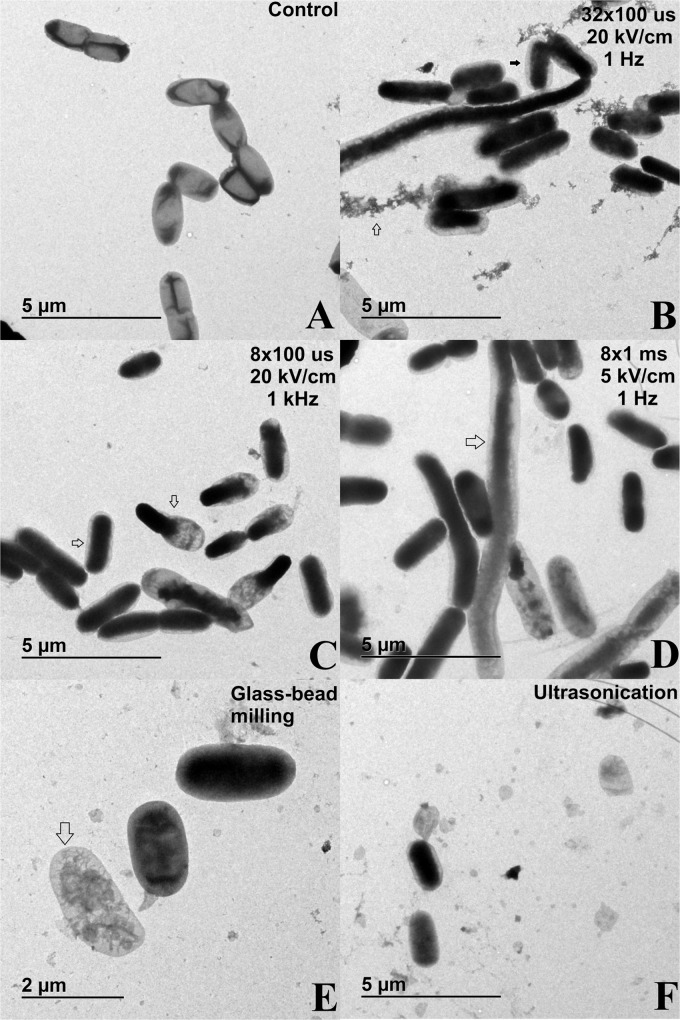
TEM images of bacterial cells (*E. coli* TOP10 pEGFP-N1) subjected to different treatment: (A) control sample – bacterial suspensions of *E. coli* TOP10 pEGFP-N1 that were not subjected to extraction methods but were otherwise treated in the same way as experimental samples; (B) electric pulses 32 × 100 μs, 20 kV/cm, 1 Hz; (C) electric pulses 8 × 100 μs, 20 kV/cm, 1 kHz; (D) electric pulses 8 × 1 ms, 5 kV/cm, 1 Hz; (E) glass–bead milling; (F) ultrasonication. Bacterial cells in exponential growth phase were incubated before and after treatment at 37°C.
